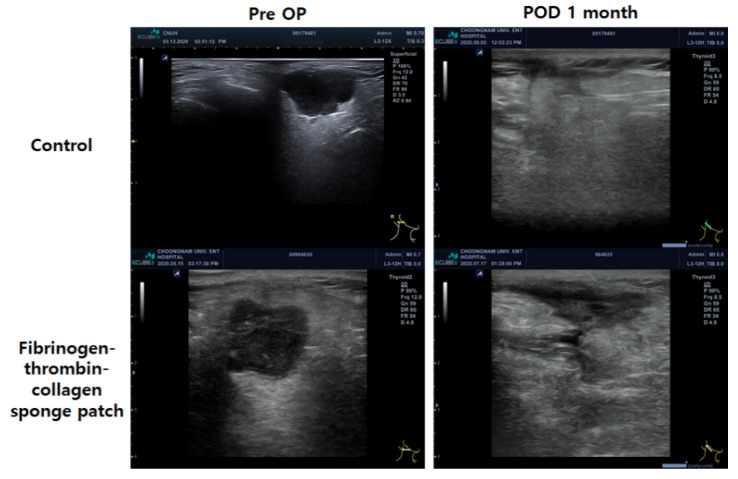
Representative preoperative and 1-month postoperative ultrasonographic images of parotid gland surgery (final diagnosis of benign parotid tumor). Preoperative transverse sonograms show well-circumscribed, multilobulated hypoechoic masses in the superficial lobe of the right parotid gland with posterior acoustic enhancement. Tumors were entirely resected through parotid surgery in both the control and fibrinogen-thrombin-collagen sponge patch groups. The 1-month postoperative transverse sonogram shows an irregularly shaped hypoechoic lesion (thickness: 0.42 cm) in the parotidectomy bed, suggestive of fibrosis. In the fibrinogen-thrombin-collagen sponge patch group, the parotidectomy defect has been replaced by markedly thicker heterogeneously hypoechoic lesions (thickness: 1.1 cm).
